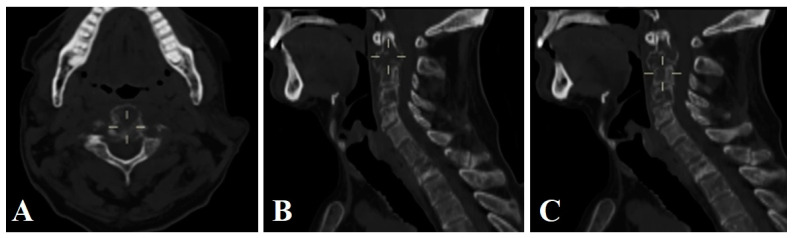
Axial (A) and sagittal (B,C) CT scans lytic lesions of cervical spine from C1 to C4, affecting the whole body of C2 and the odontoid process.
